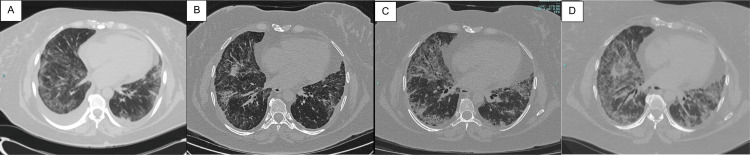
Timeline of CT chest imaging (A) CT image of the chest at week 4 revealed low-volume bilateral pleural effusion, mild pericardial effusion, and patchy areas of subpleural ground glass opacities and reticulation. (B) CT image of the chest at week 18 showed minimal pleural thickening and small pericardial effusion with mild bilateral subpleural dominant ground glass opacities, indicating improvement compared with the study at 4 weeks. (C) CT image of the chest at week 76 showed worsening patchy and confluent ground glass opacity with septal thickening. (D) CT image of the chest at week 92 showed further progression of ground glass opacities and septal thickening CT: computed tomography
